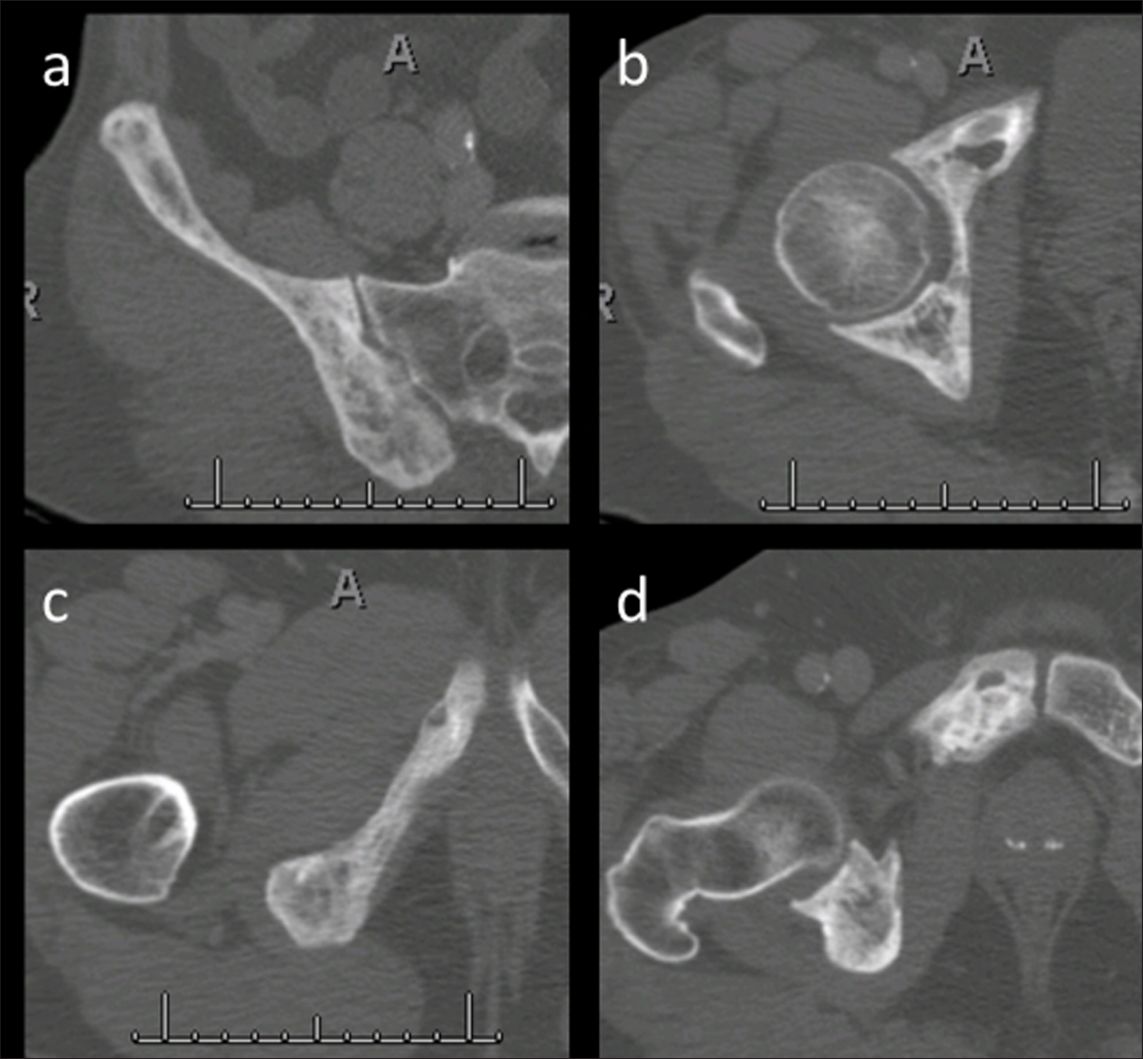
The axial CT images show cortical thickening, trabecular coarsening, and expansion in the right iliac bone (a), acetabulum (b), inferior pubic ramus (c), and pubic bone (d) due to Paget’s disease.
